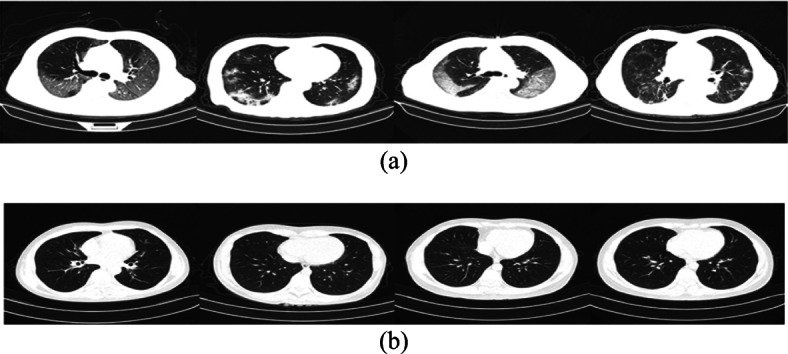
Example chest CT images from the COVIDx-CT dataset, (a) COVID-19 cases, and (b) Normal cases
